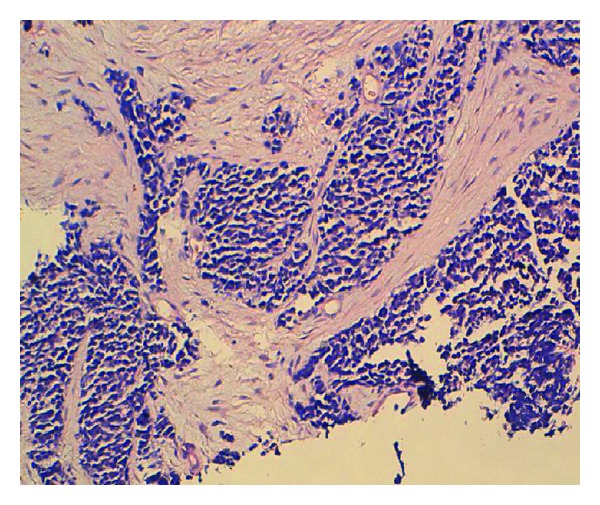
CT-scan-guided TCB slide of a pancreatic mass in a 56 year-old man: cytomorphological features at higher magnification view: small, round tumor cells with scanty cytoplasm, which showed extensive nuclear molding. The nuclei of the tumor cells were round to oval and had salt and pepper type chromatin pattern.
